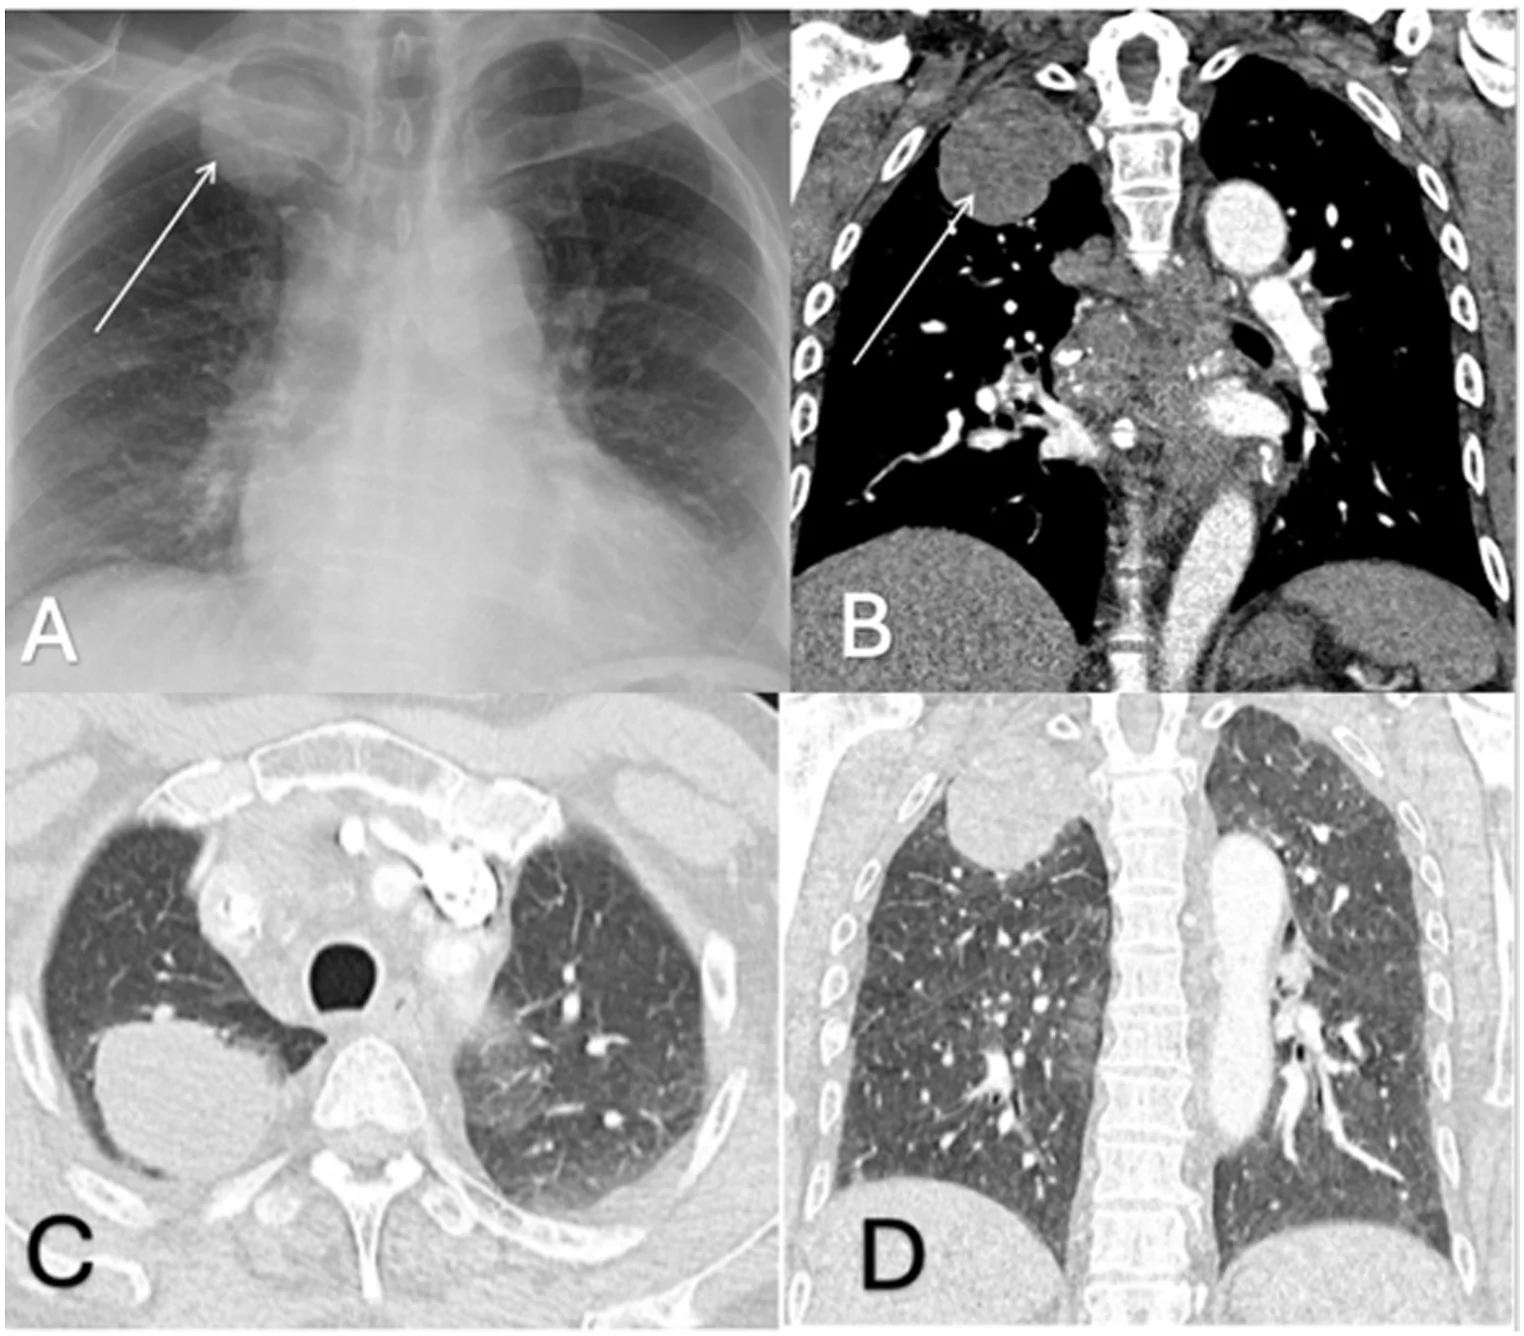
Interval imaging at six months: 2A: PA chest radiograph showing progression to right upper lobe apical mass (5.1 cm × 4.9 cm) (white arrow). Interval reduction in cardiac silhouette size. No new parenchymal features of pulmonary TB. 2B: 3 mm coronal soft tissue reconstructed contrast-enhanced CT showing right apical soft tissue mass (white arrow) with bilateral hilar and paratracheal calcified lymph nodes. 2C (axial) and 2D (coronal): 3 mm axial lung reconstructed contrast-enhanced CT showing no CT evidence of active TB.
